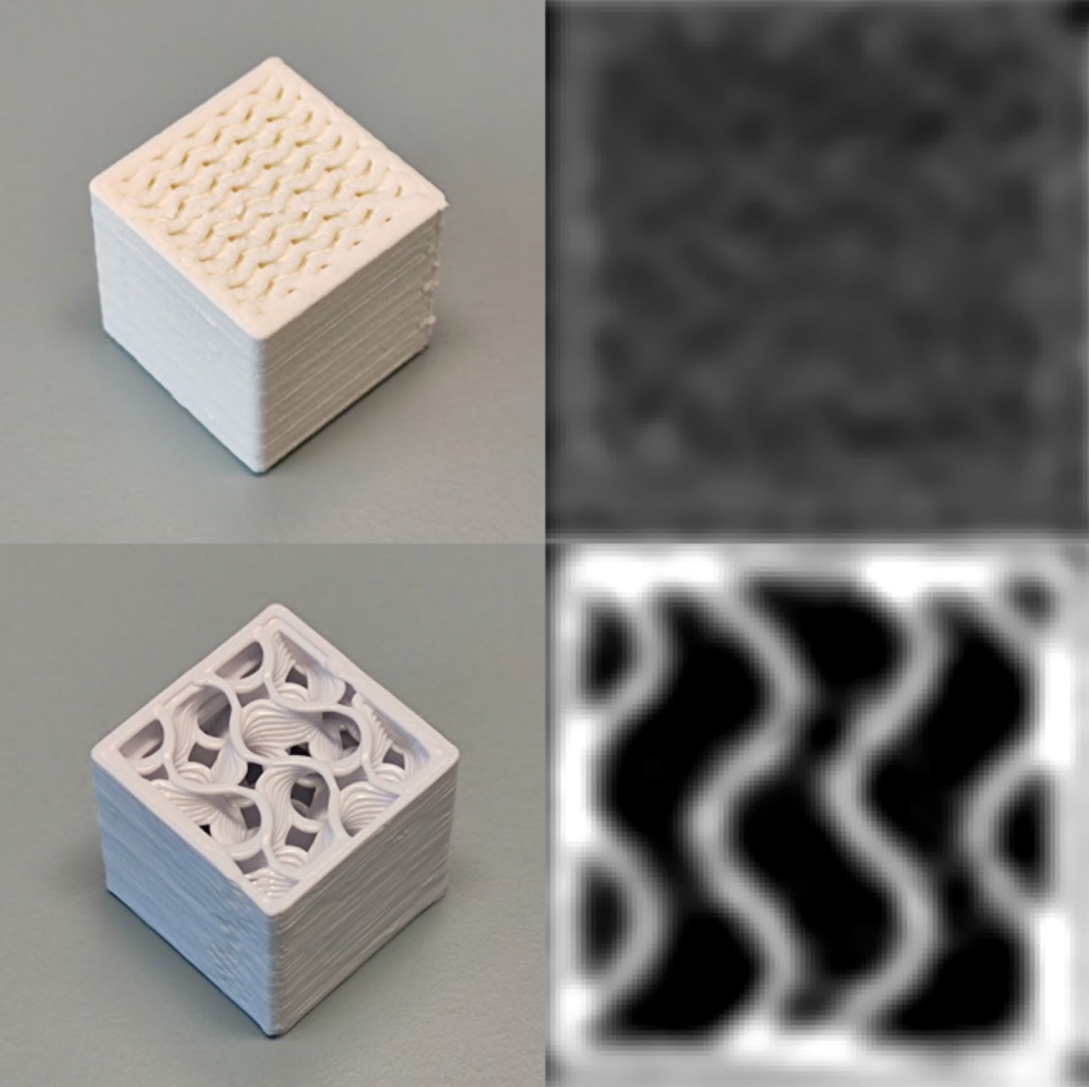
Photographs (left) and central CT slices (right) of test cubes with equivalent RED of 0.24 printed with Raise3D Pro 2 0.8 mm nozzle using PLA-LW at 260 °C at 60% in-fill and a flow of 36% (top) and PLA + at 20% in-fill (bottom), shown with identical window settings (width: 1000, level: -500)
